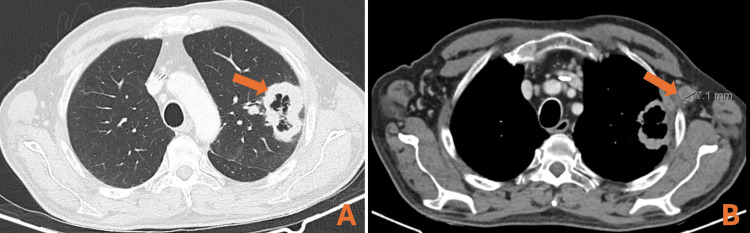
Restaging CT scan showing (A) increased lung lesion and the development of (B) left axillary lymph node CT: computed tomography
